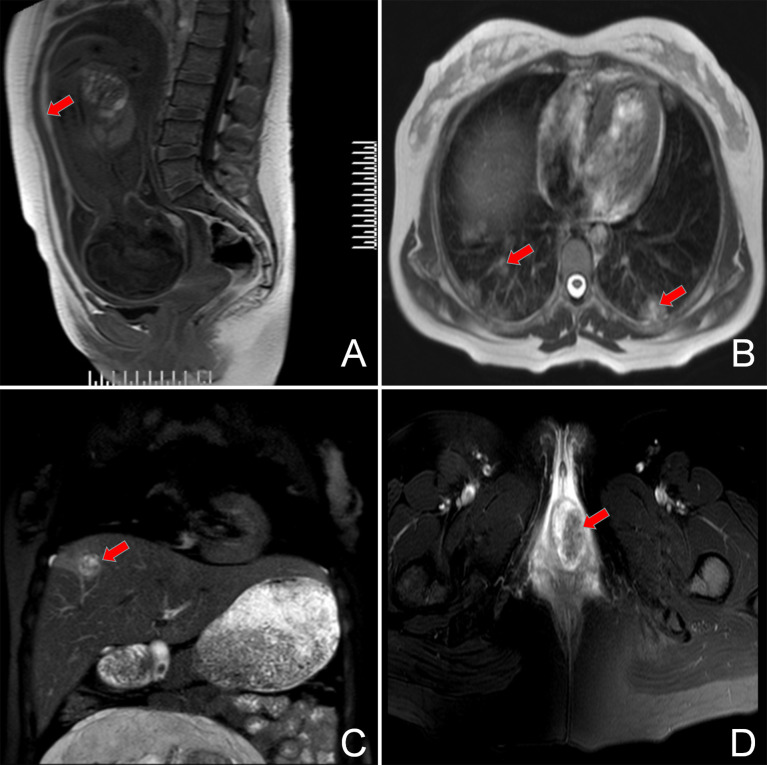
MRI film showing the original lesion in the anterior uterine wall (A) and the metastasis lesion in the bilateral pulmonary tissue (B), right lobe of the liver (C), and the vagina (D).
